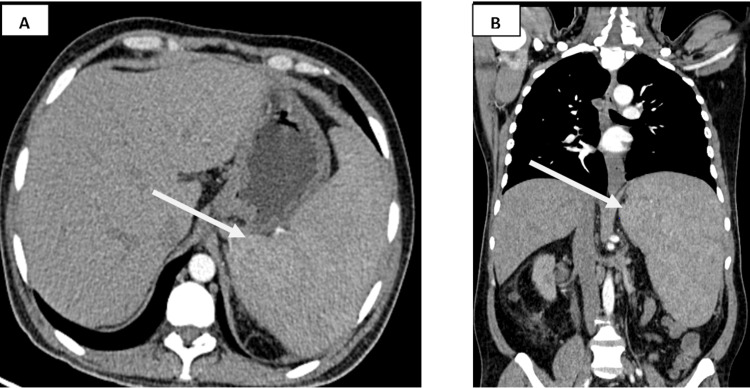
(A) Axial and (B) coronal reconstructed contrast-enhanced CT image in the arterial phase showing gross splenomegaly.
